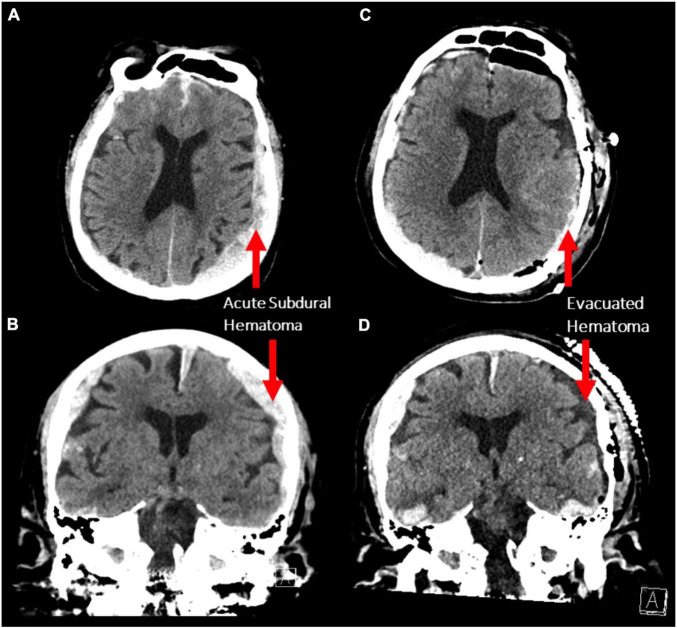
(A,B) Axial and coronal non-contrast CT scans demonstrating bilateral acute subdural hematoma with a larger mass effect on the left side (maximum thickness 1.5 cm). (C,D) The same scan sequences after decompressive craniotomy demonstrating evacuation of the left subdural hematoma.
